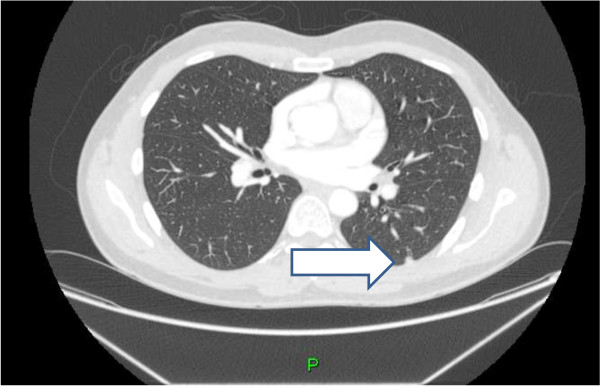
CT image of a lung nodule.
